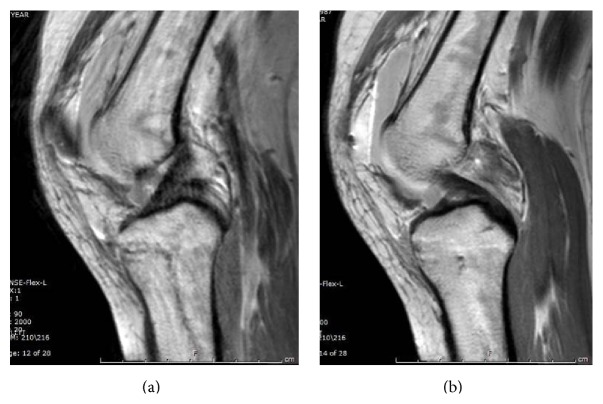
Initial sagittal MRI scans showing intact anterior cruciate ligament (a) and posterior cruciate ligament (b).
